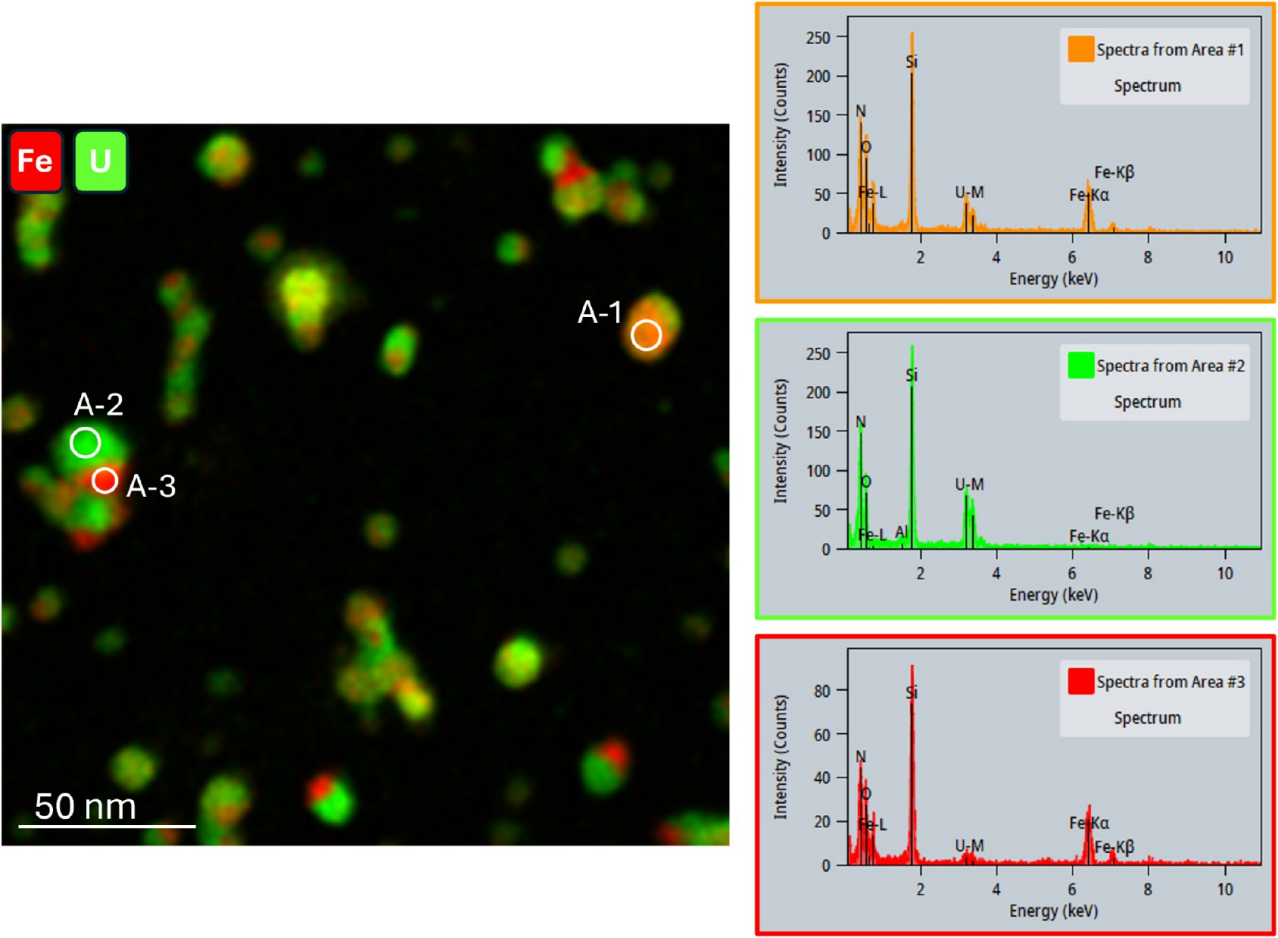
Mosaic X-ray map of elemental Fe (red) and U (green) from
